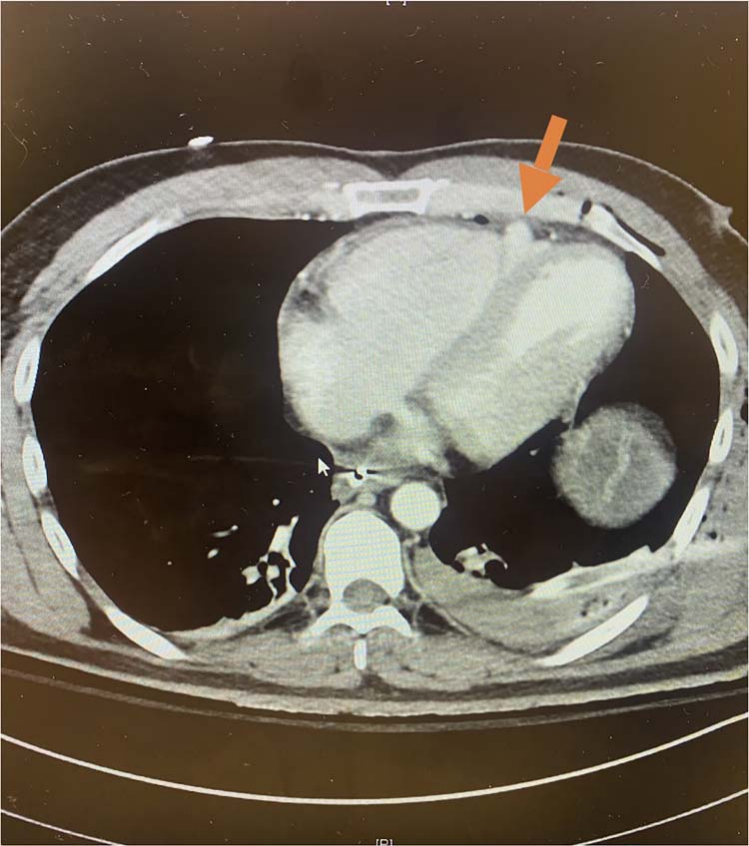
Axial CT imaging demonstrating pseudoaneurysm of right ventricular free wall rupture.
